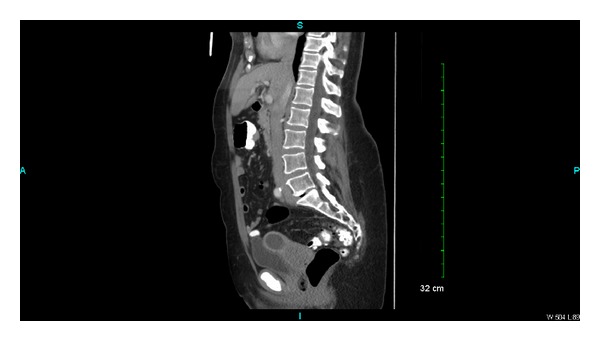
CT scan of abdomen and pelvis showing fluid-filled and distended uterine endometrial cavity with free fluid in the posterior cul-de-sac.
